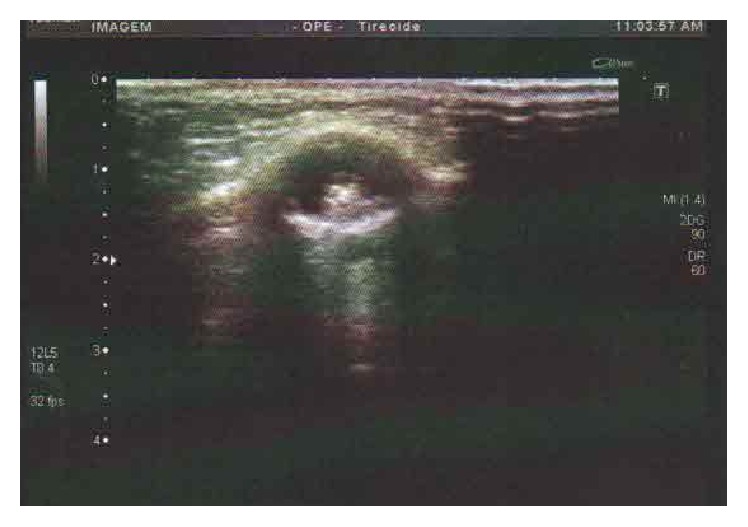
Sonography shows an image suggestive of thyroglossal duct cyst containing debris and an eccentric small hyperechoic solid area.
